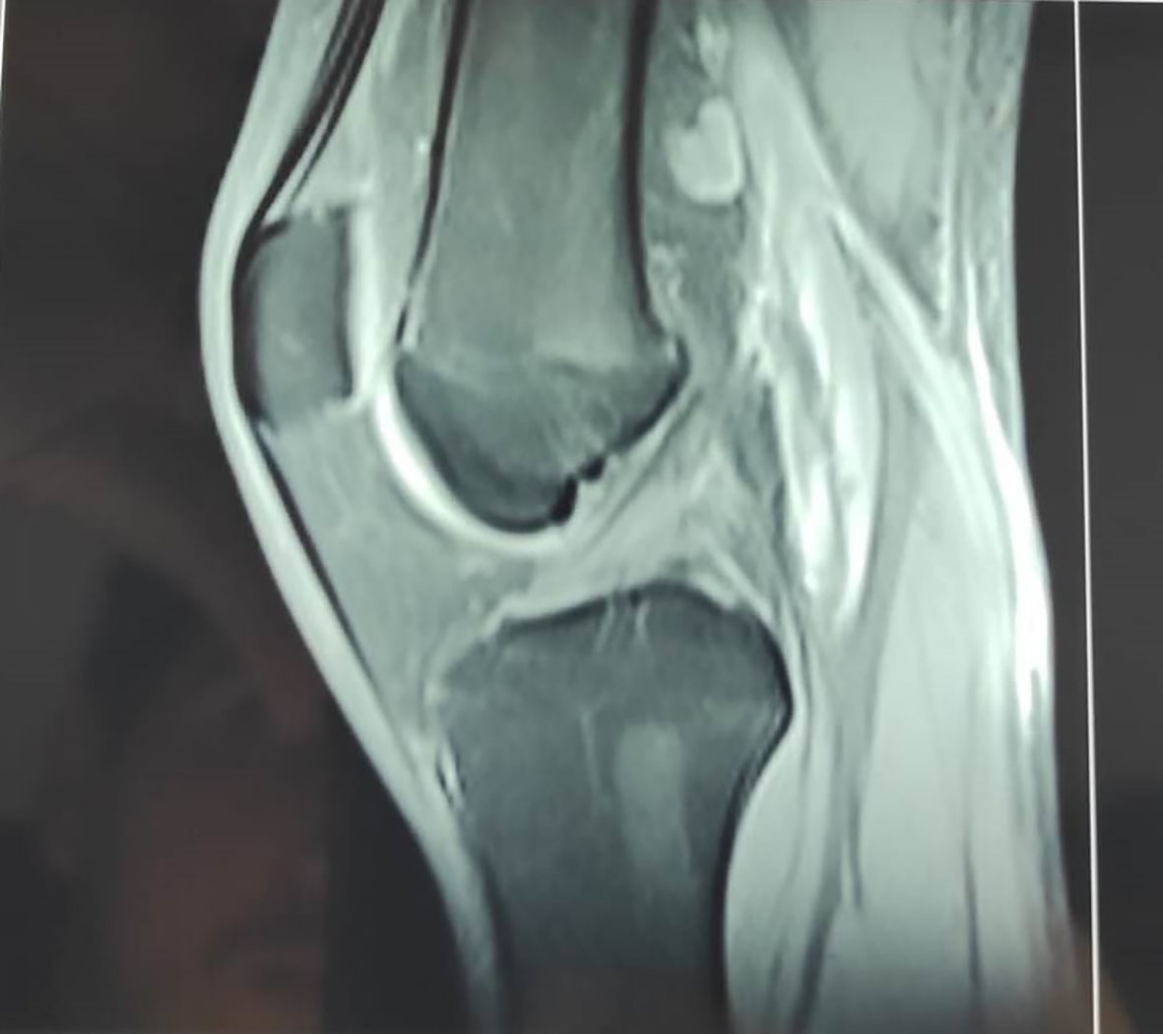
A control MRI demonstrated complete disappearance of the previously described mass with no joint effusion.
